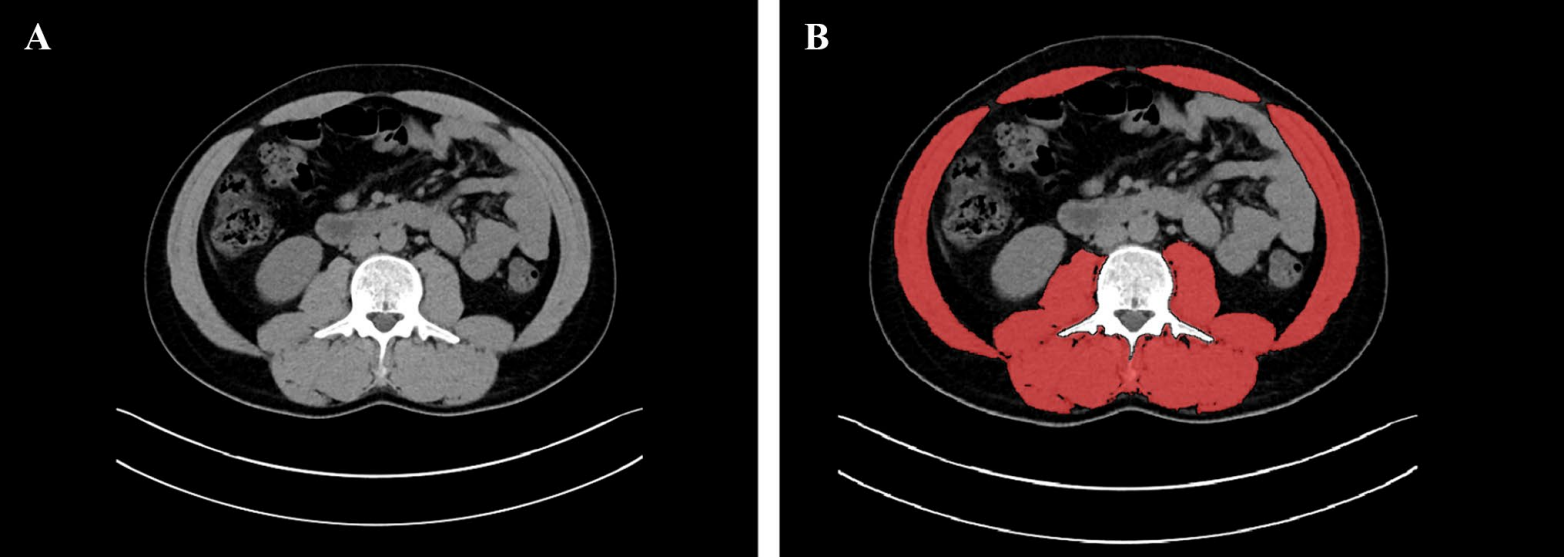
A Left—axial slice of non-contrast CT abdomen at the L3 vertebral level. B Right—all skeletal muscle at this level is highlighted in red
